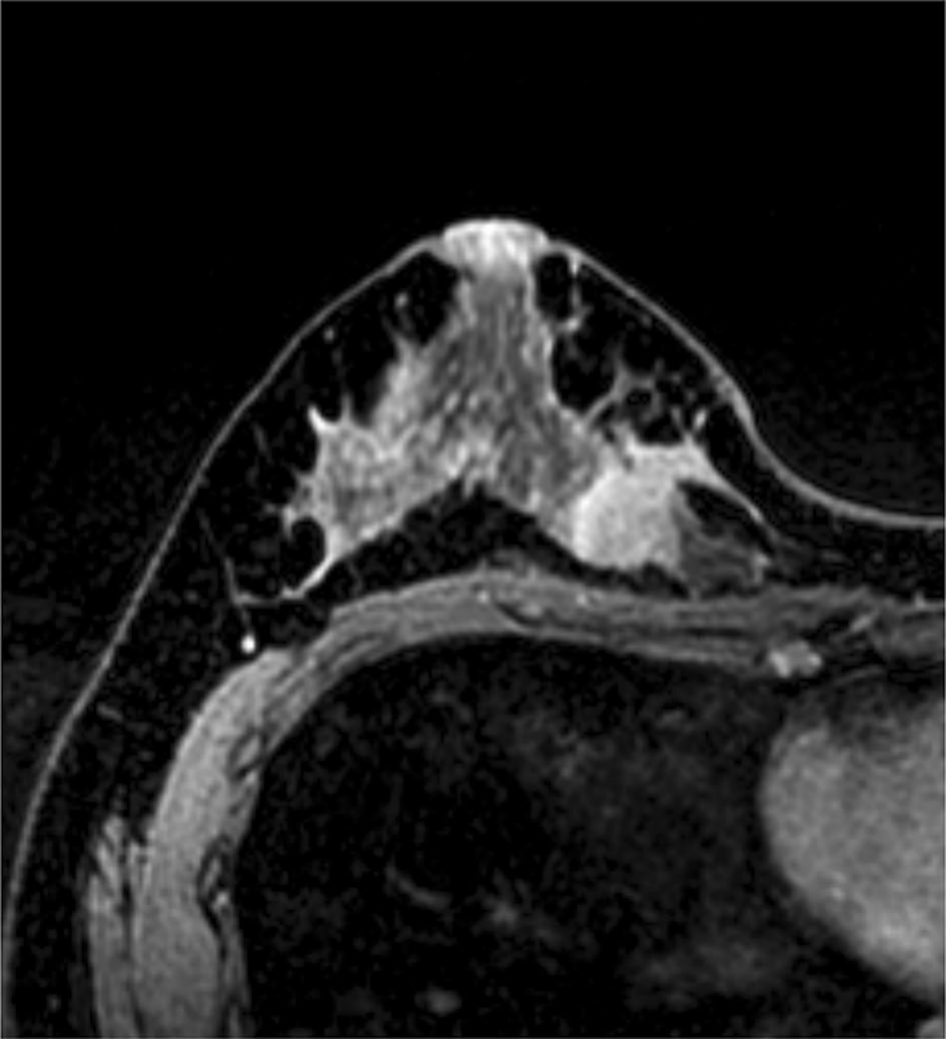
Contrast-enhanced magnetic resonance imaging in case 5 revealed a partially enhanced irregular mass of 3.2 cm in the upper-inner area of the right breast
